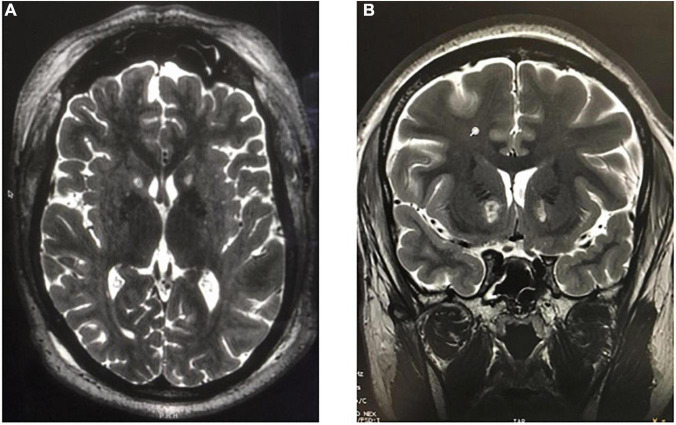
MR-guided focused ultrasound capsulotomies (MRgFUS). (A) Axial T2-weighted and (B) Coronal T2-weighted MRI after bilateral MRgFUS capsulotomy.
